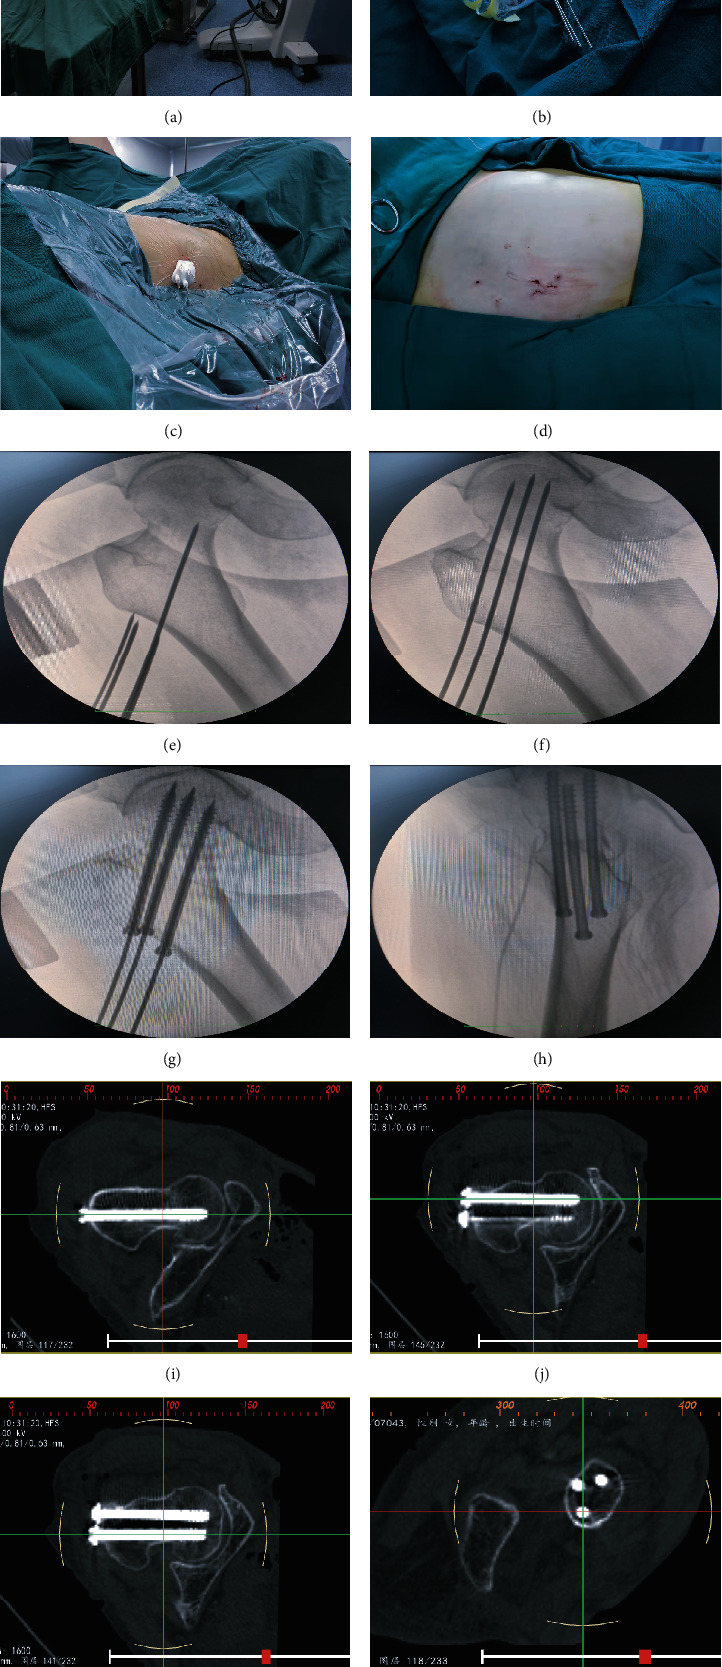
The accuracy of computer-aided design and 3D printing technology for guide plate production. (a–d) The surgical procedure of 3D-printed guide plate-assisted percutaneous needle insertion and cannulated screw placement. (e–h) C-arm X-ray fluoroscopy was used to observe the cannulated compression screw placement. (i–l) Immediate postoperative CT was performed to evaluate fracture reduction and the position of the three cannulated compression screws.
